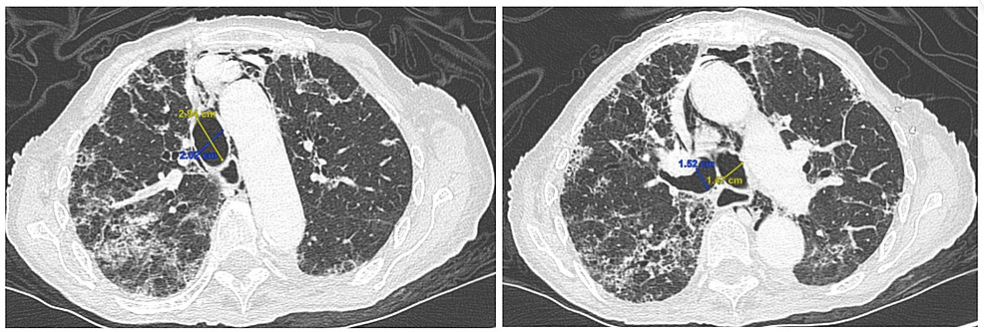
Computed tomography (CT) of the chest obtained six months after COVID-19 infection highlighting the increase in sagittal (yellow) and coronal (blue) diameters of the trachea (left) and at the level of tracheal bifurcation (right) highlighting the diameter of the right and left bronchi COVID-19: coronavirus disease 2019
